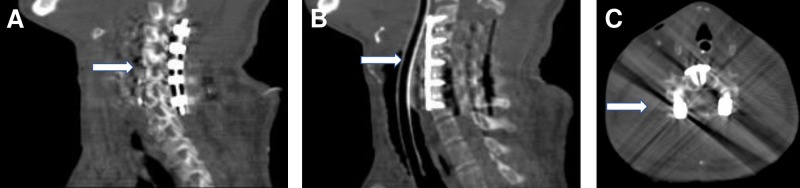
Cervical Spine Computed Tomography After Second Posterior Cervical Decompressive Surgery Postoperative sagittal computed tomography shows bilateral C3-C7 lateral mass screws (A) placed after posterior decompression, and anterior cervical screw-plate instrumentation (B) placed after anterior decompression surgery. Postoperative axial computed tomography (C) shows placement of both posterior and anterior instrumentation.
